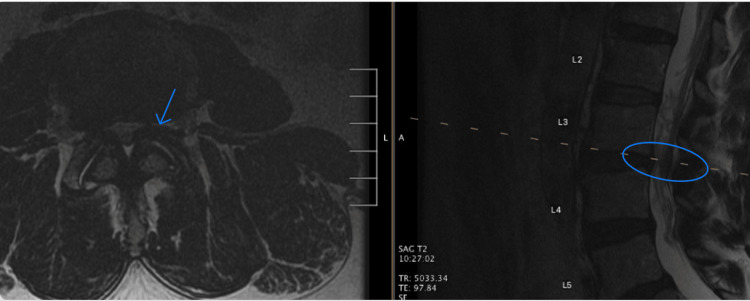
MRI showing Floseal aggregate in the epidural space at the decompressed level - one week postoperative. Shown in blue is the Floseal aggregate, which was originally misdiagnosed by the neuroradiologist as an epidural abscess. MRI, magnetic resonance imaging
